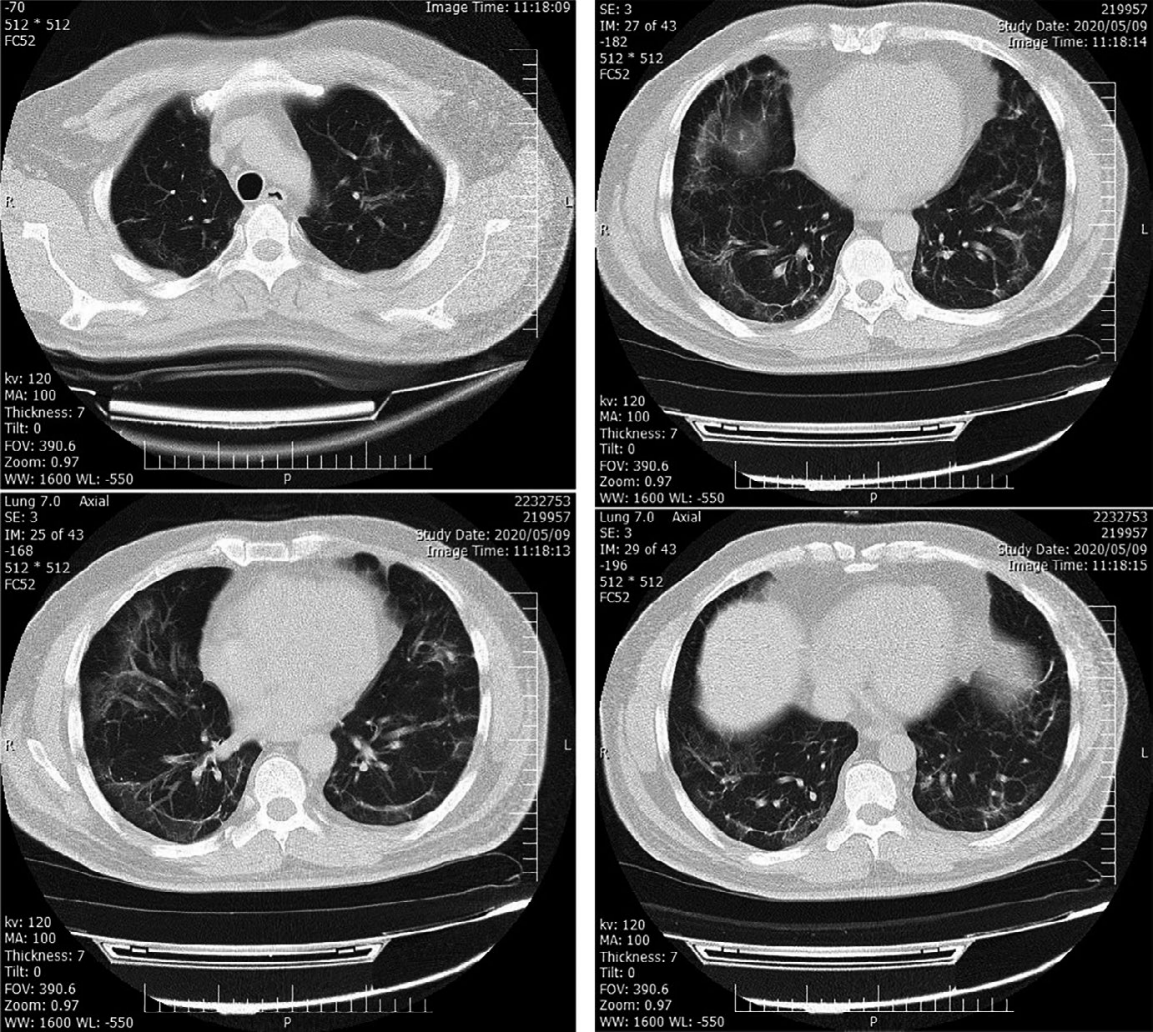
Chest CT scan at first admission
